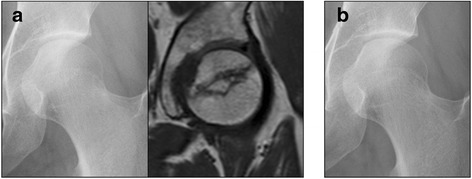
Case 2. Twenty-seven years old woman with NOFH. a Anteroposterior radiograph and magnetic resonance imaging (T1WI) at the first examination showing NOFH. After diagnosis, teriparatide was administered. b The femoral head showed no progression of collapse for one year and 8 months
